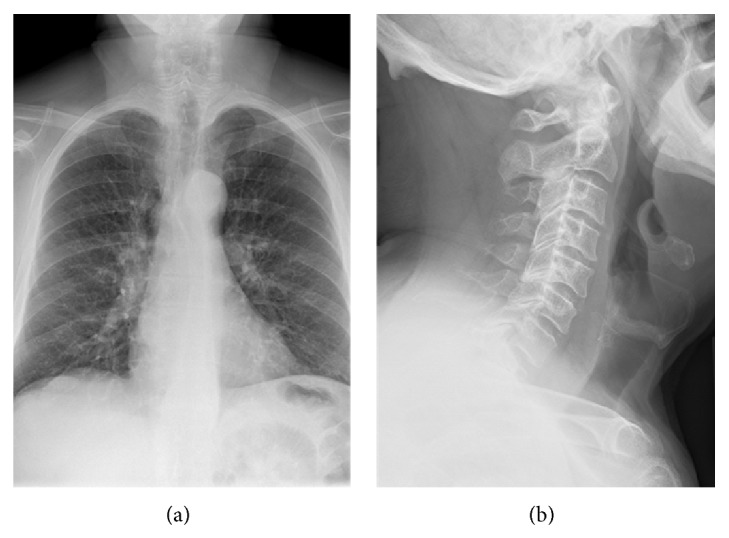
PA chest (a) and lateral neck (b) radiographs obtained day of suspected aluminum tab aspiration fail to demonstrate a metallic foreign body. There is no evidence for supportive findings on chest radiographs such as atelectasis or air-trapping.
